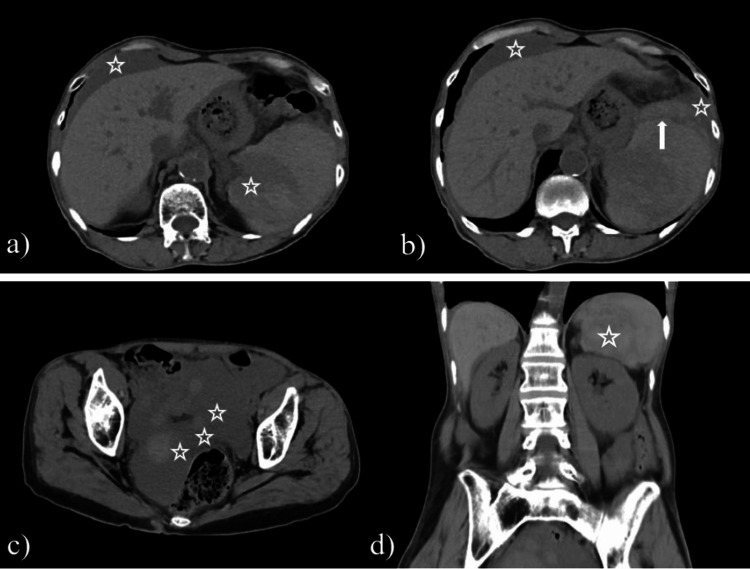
CT scan images a) Markers indicate hemoperitoneum around the liver and the spleen; b) Arrow indicates the location of the rupture and star markers indicate hemoperitoneum around the liver and spleen; c) Hyperdense free fluid in the Douglas Pouch; d) Coronal image, star marker indicates the location of the ruptured spleen and the surrounding perisplenic hematoma.
